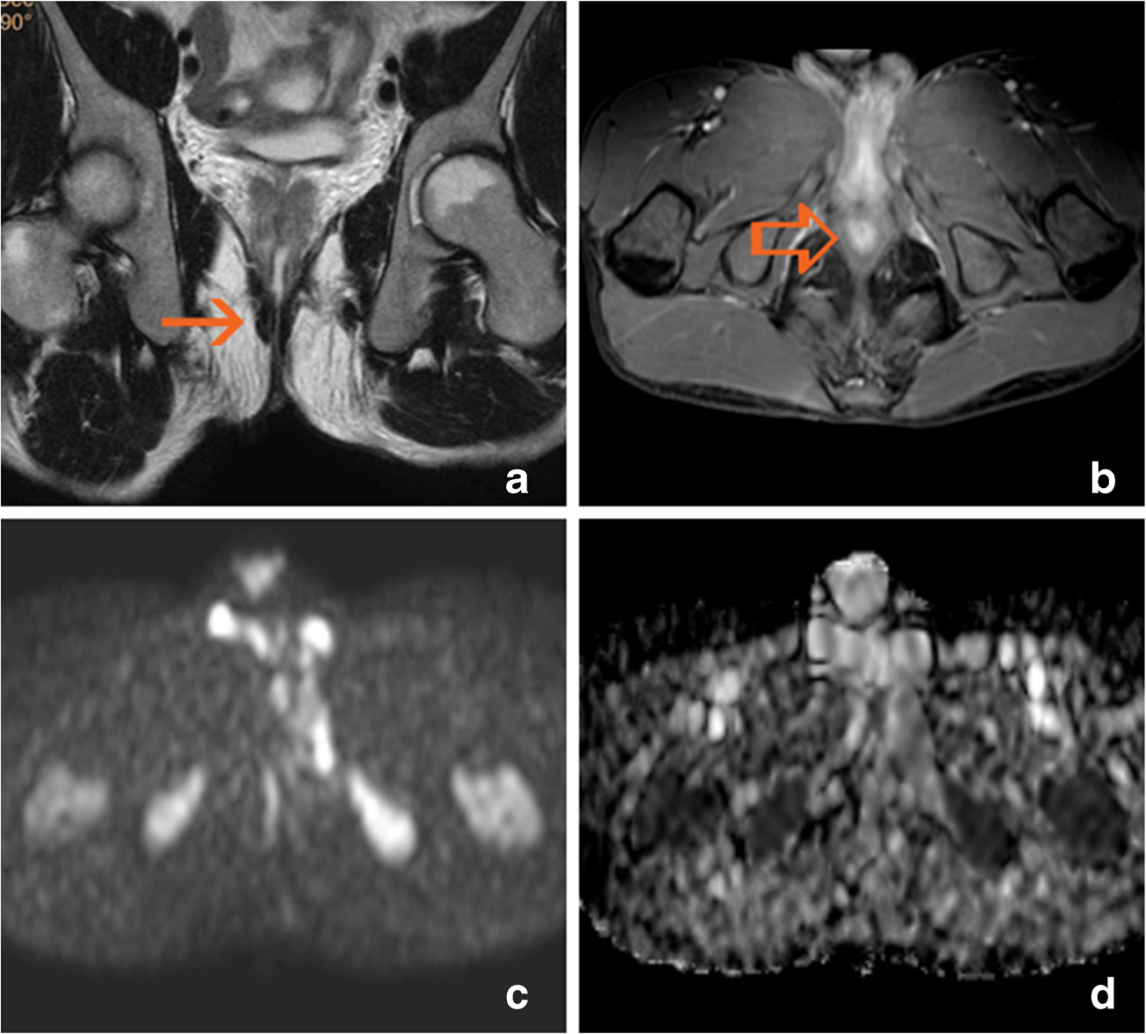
Anal fibrous stenosis. The internal anal sphincter shows hypointensity on T2-weighted sequences and diffuse enhancement after gadolinium administration (arrows); the native diffusion-weighted image and the ADC map show hypointensity. a Oblique-coronal T2-weighted image. b Post-gadolinium oblique-transverse fat-supressed gradient echo T1-weighted image. c Native oblique-transverse native diffusion-weighted image with 800 s/mm^2^ b factor. d ADC map
